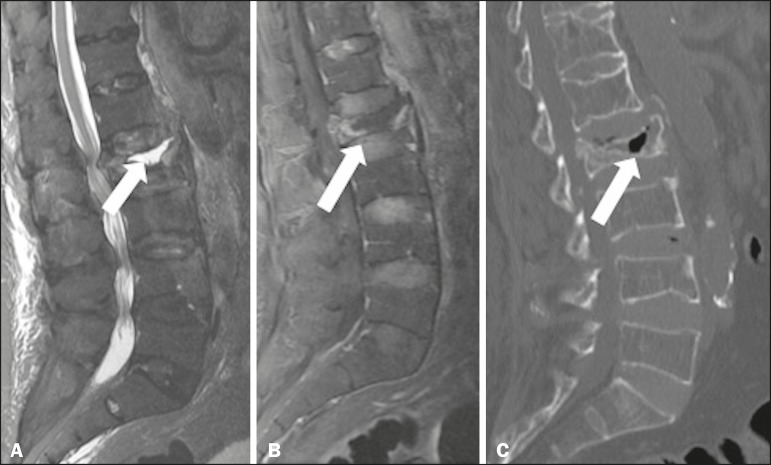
Gadolinium contrast-enhanced, fat-saturated T1- and T2-weighted MRI
